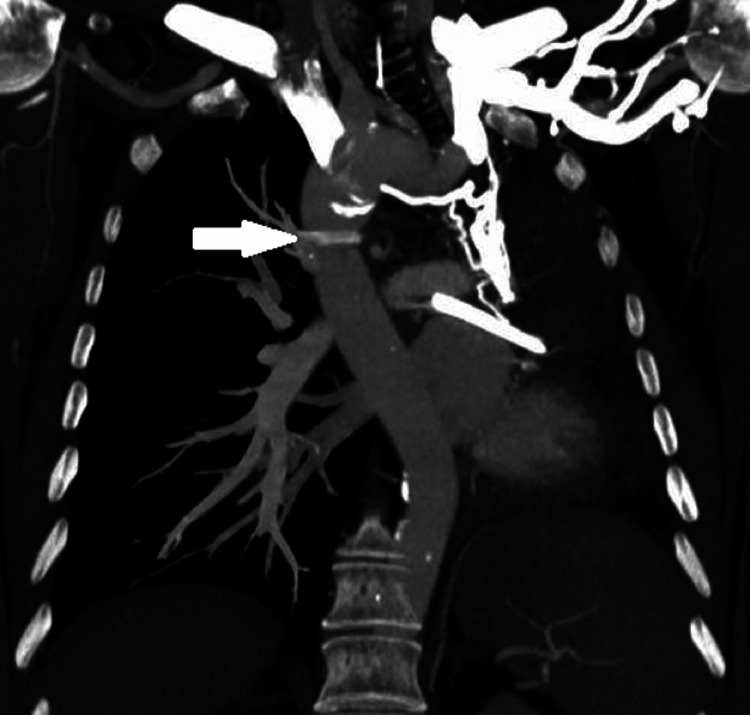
Coronal soft tissue window. Maximum intensity projection (MIP) computed tomography angiographic image demonstrates a right-sided aortic arch (arrow).
